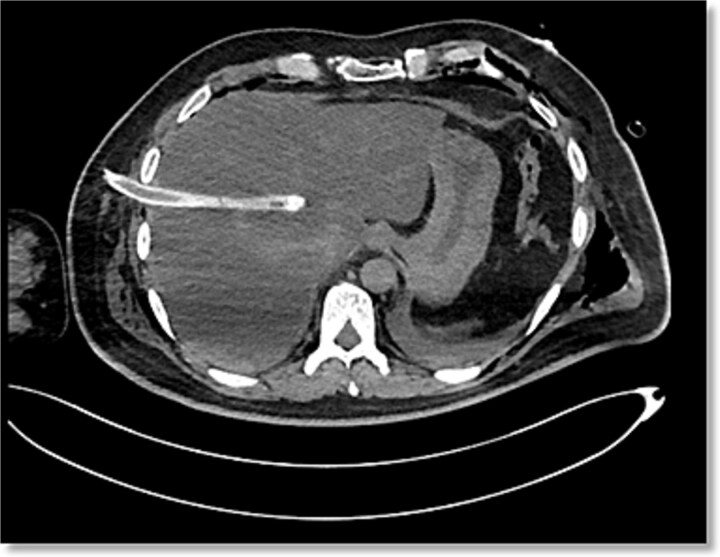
Non-contrast abdominal CT, axial view, demonstrating penetrating hepatic trauma caused by iatrogenic chest tube placement involving hepatic segments VII, VIII, and I.
